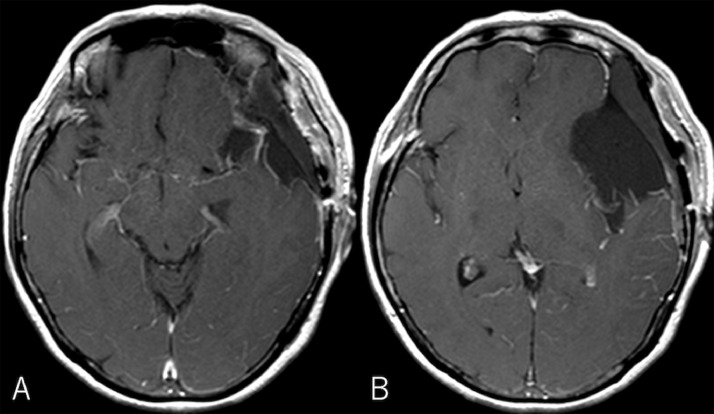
Post-contrast axial magnetic resonance images showing a comfortable resection of the tumor.
